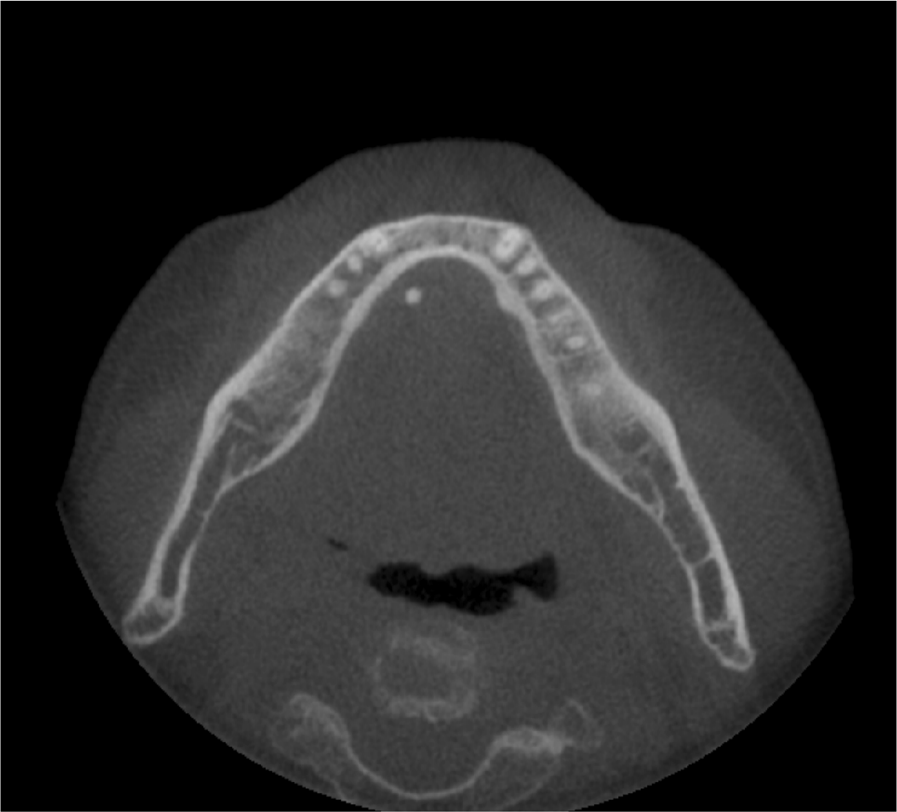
Salivary stones measuring 3 × 4 mm are detected in the right submandibular gland with cone-beam CT
